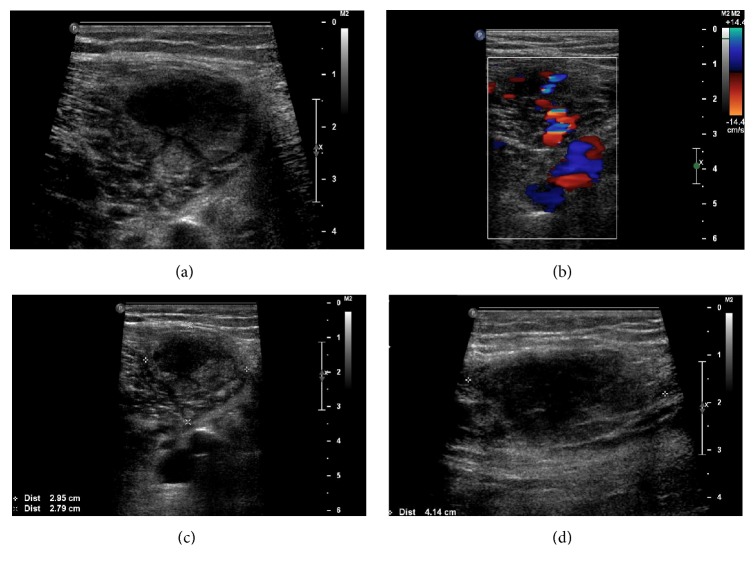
Gray scale images in transverse (a, c), longitudinal (d), and color Doppler (b) of the distal right thigh of a 51-year-old male with bilateral lower extremities pain and swelling. Images demonstrate area of heterogeneous echogenicity with internal flow in the distal right thigh. Initial interpretation was that the findings might represent muscular hematoma with possible active bleeding from overanticoagulation.
